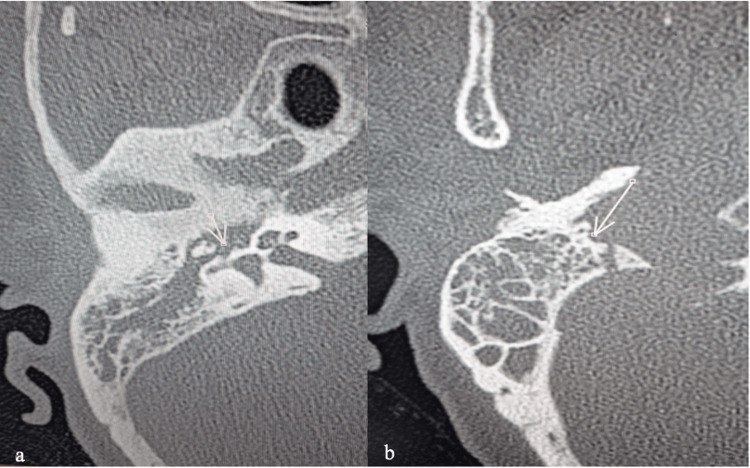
Skull base CT. (a) Axial image showing bone lysis of the anterior wall of the tympanic segment of the facial nerve canal. (b) Axial image showing bone lysis of the anterior wall of the mastoid CT: computed tomography
